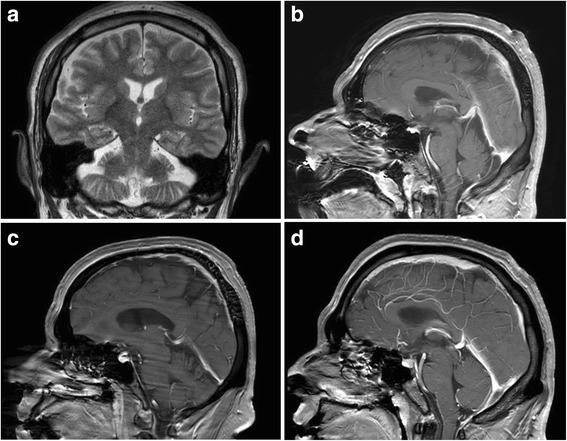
Brain MRI of the three affected siblings. a T2-weighted coronal scan of the older male patient (Fig. 1b: II-1) demonstrates cerebellar hypoplasia/atrophy with the impression of a cleft in the lateral cerebellar hemisphere. Note that the brainstem volume is preserved; (b, c, d) T1-weighted sagittal scans with contrast of all three siblings (Fig. 1b: II-1, II-2 and II-3, respectively) show slight hypoplasia/atrophy of the cerebellar vermis. Also, there is downsloping splenium of the corpus callosum
